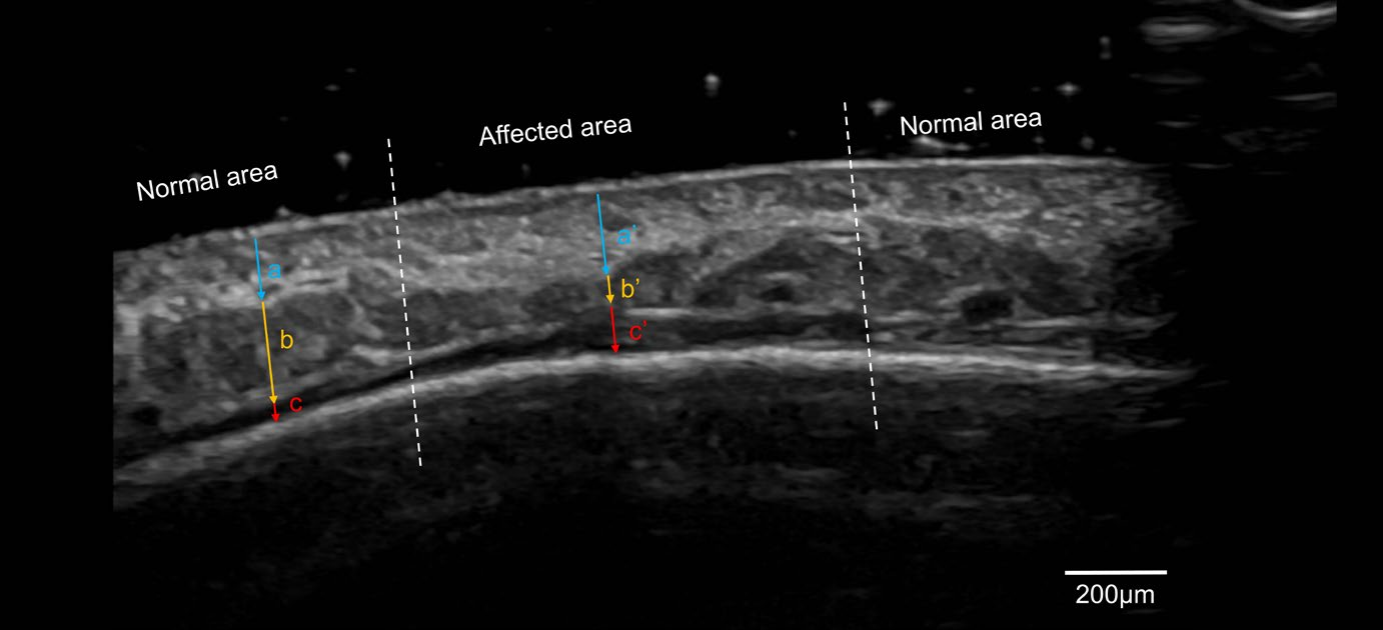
Ultrasound images of the patient's forehead, showing both normal and affected regions. (a) and (a’) correspond to the dermal thickness in the normal and affected regions, measuring 1.4 and 1.8 mm, respectively. (b) and (b’) represent the thickness of the subcutaneous fat layer in the normal and affected regions, measuring 2.0 and 0.8 mm, respectively. (c) and (c’) depict the thickness of the frontalis muscle and the subfascial space in the normal and affected regions, measuring 0.4 and 0.9 mm, respectively.
